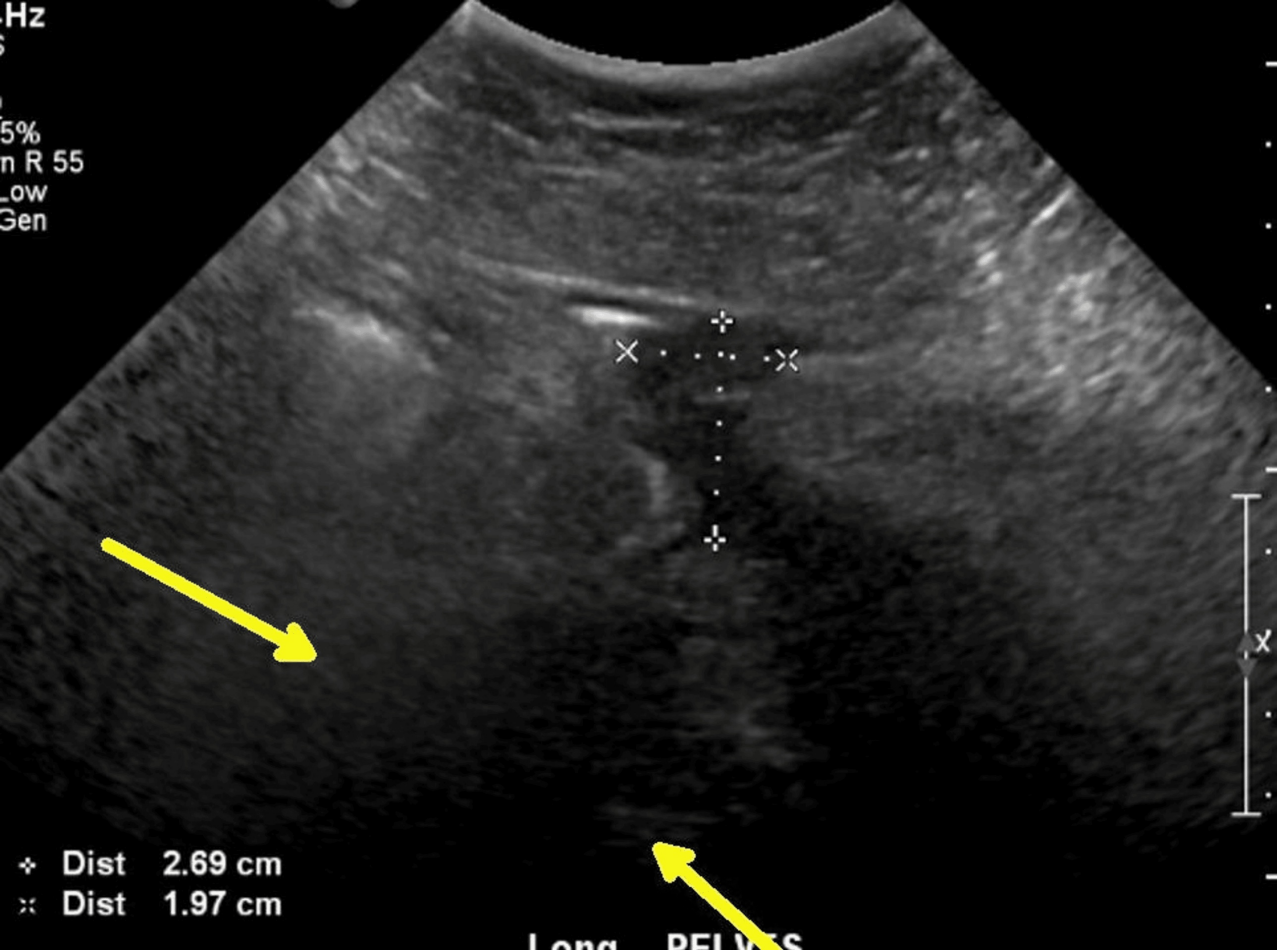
Transabdominal ultrasound (day 28 after initial admission and 13 days after cesarean delivery) showing the complex fluid collection anterior to the uterus between the cursors, measuring 2.69 cm x 1.97 cm. The region of the uterus is below the acoustic shadowing at the yellow arrows.
